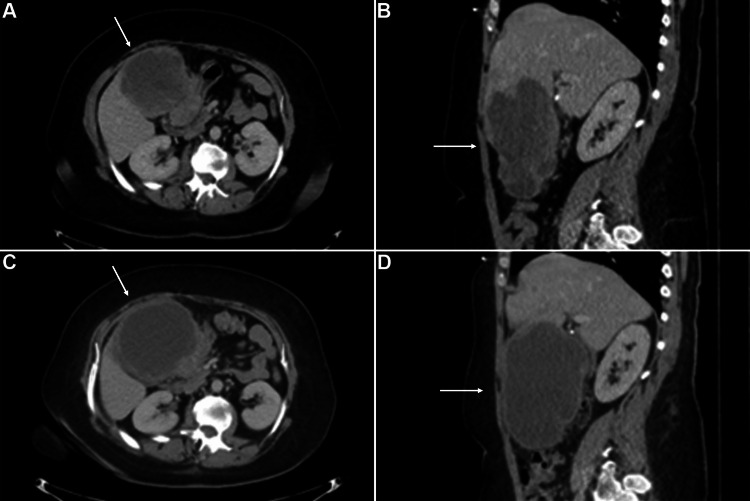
CT images of the liver lesion A, B) CT images (A axial, B sagittal) prior to chemotherapy demonstrated a 14.3 x 11.6 cm mass (arrows) originating from segment IVb of the liver. C, D) On repeat CT scan (C axial, D sagittal) after neoadjuvant chemotherapy, the tumor was more encapsulated with a notable increase in central tumor necrosis.
